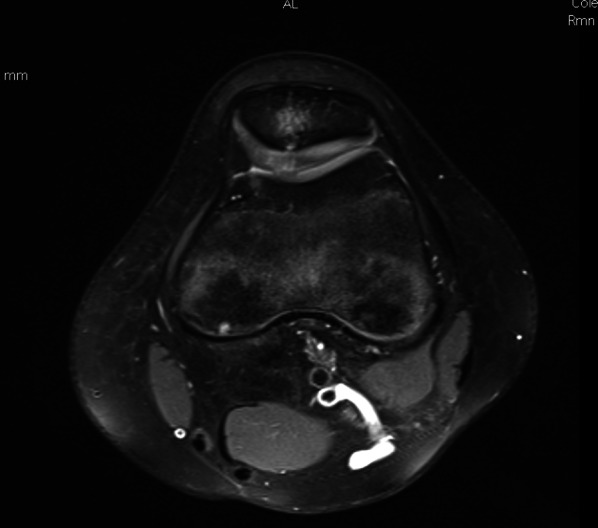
Left knee; MRI axial view; gross full-thickness erosion of the articular cartilage of the patellar ridge, with subchondral bone distress of an area of the patellar body characterized by edema with a small dystrophic cystic area. The picture overlaps with femoro-patellar dysplasia of the shallow femoral trochlea
